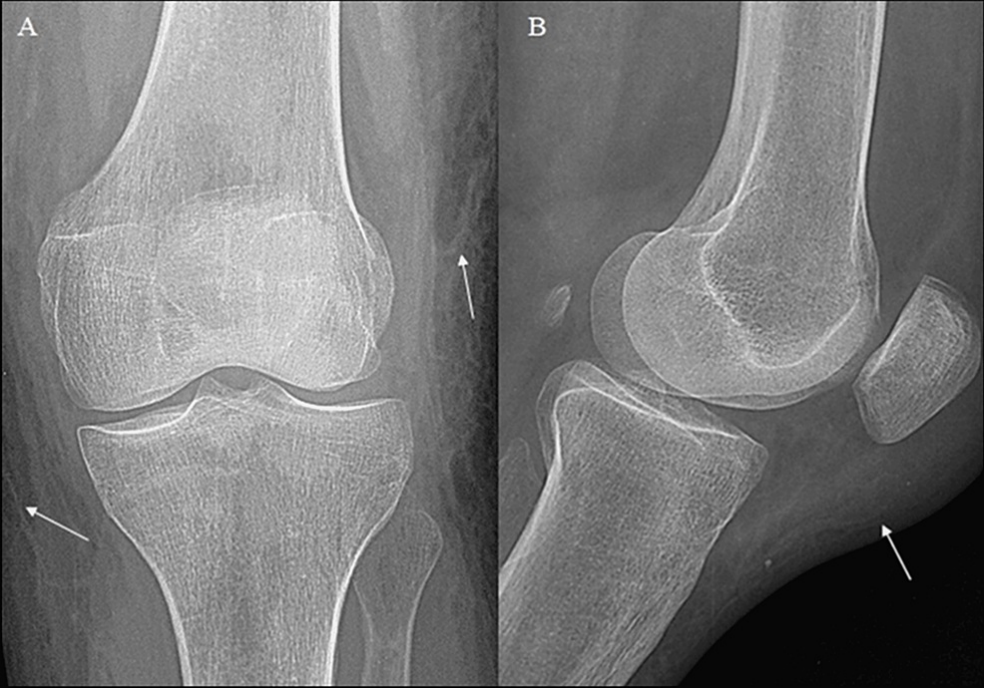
Antero-posterior (A) and lateral (B) plain radiography of the left knee depict a soft tissue swelling (arrows, A and B) predominant in the prepatellar aspect of the knee (arrow, B), with no evidence of bone or articular lesions, nor signs of intra-articular effusion.
